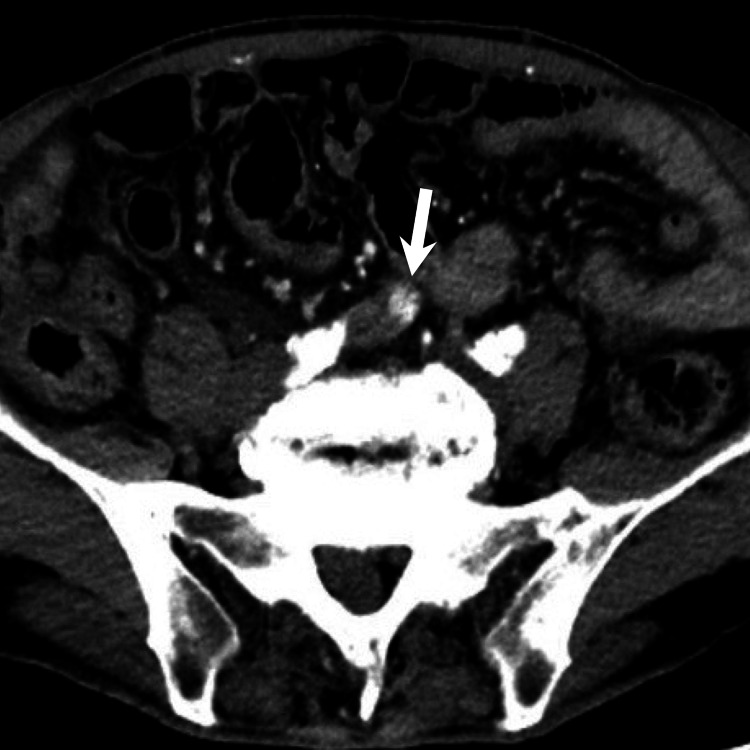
An arterial phase image of multiphase CT angiography reveals an enhanced lesion measuring 1 cm in the small bowel, corresponding to the nidus of the arteriovenous malformation (AVM) (white arrow).
